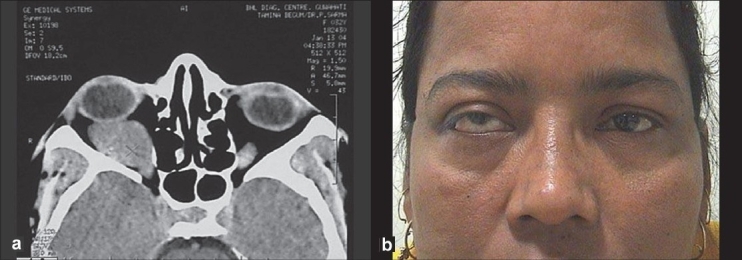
(a) CT scan of the right orbit (axial view) showed an intraconal mass lesion of the right orbit (b) clinical view of the patient (preoperative)
